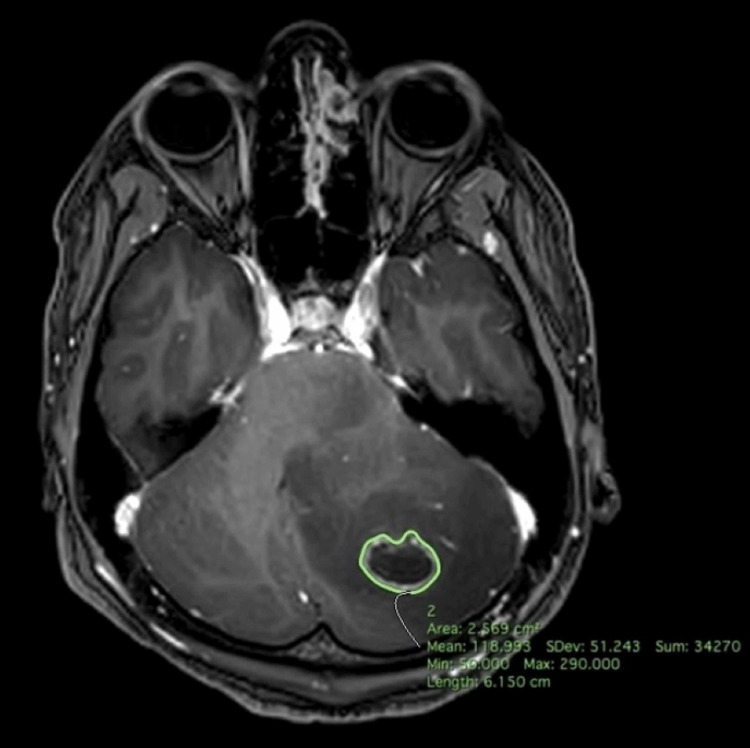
Preoperative T1-weighted MRI - Horos ROI segmentation Preoperative T1-weighted MRI demonstrating Horos region of interest (ROI) segmentation function with 4.2509 cm^3^ mass of the left cerebellum circumferentially outlined.
